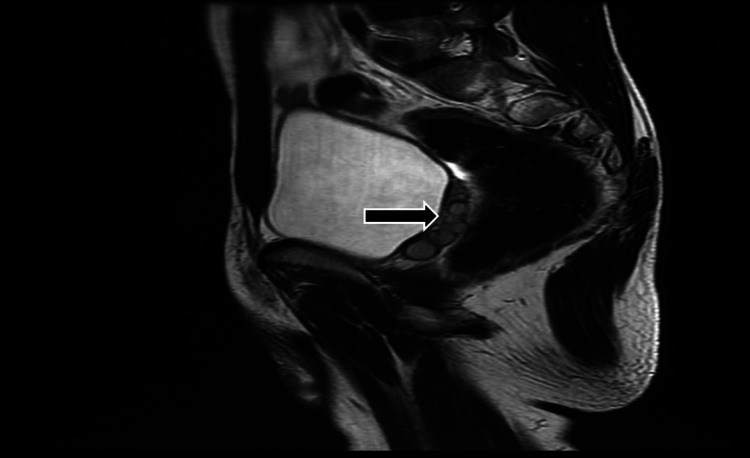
Magnetic resonance imaging (sagittal T2) showing hypointense left seminal vesicle cysts on T2 (arrow)
